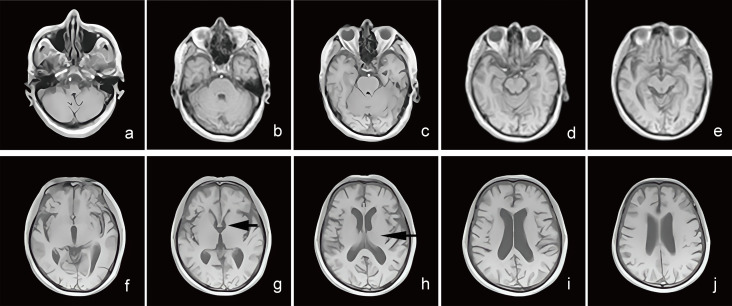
Brain MRI T1WI sequence imaging of MCI patients. (a–j) The
